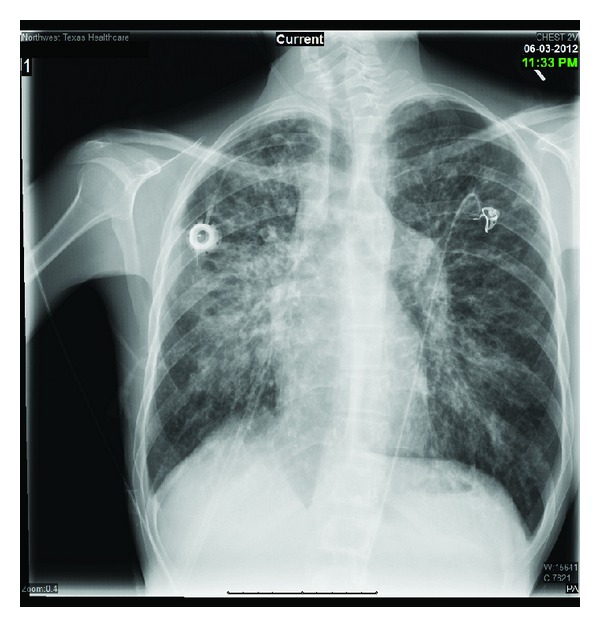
Admission chest X-ray showing right perihilar alveolar infiltrates superimposed on chronic pulmonary infiltrates.
